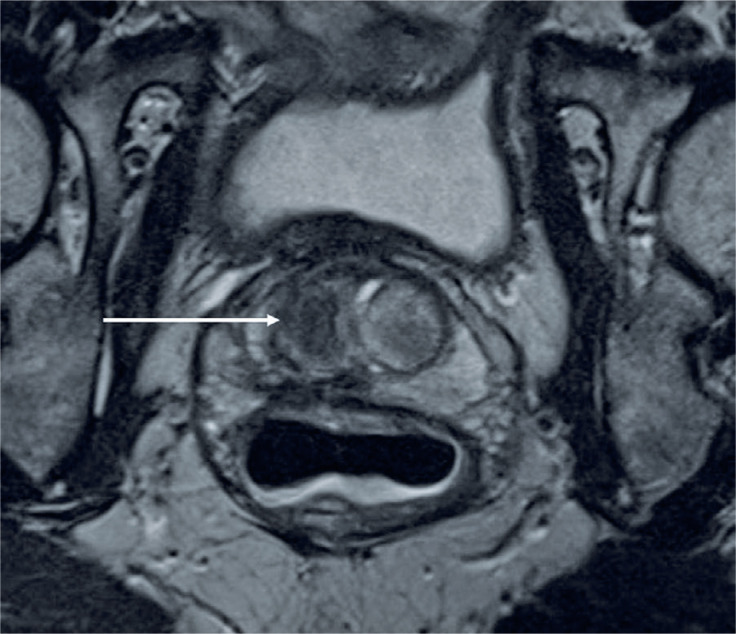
Magnetic resonance imaging (T2-weighted sequence) performed 367 days after irreversible electroporation shows no suspicious lesions. The ablation area appears with marked hypointensity (white arrow)
